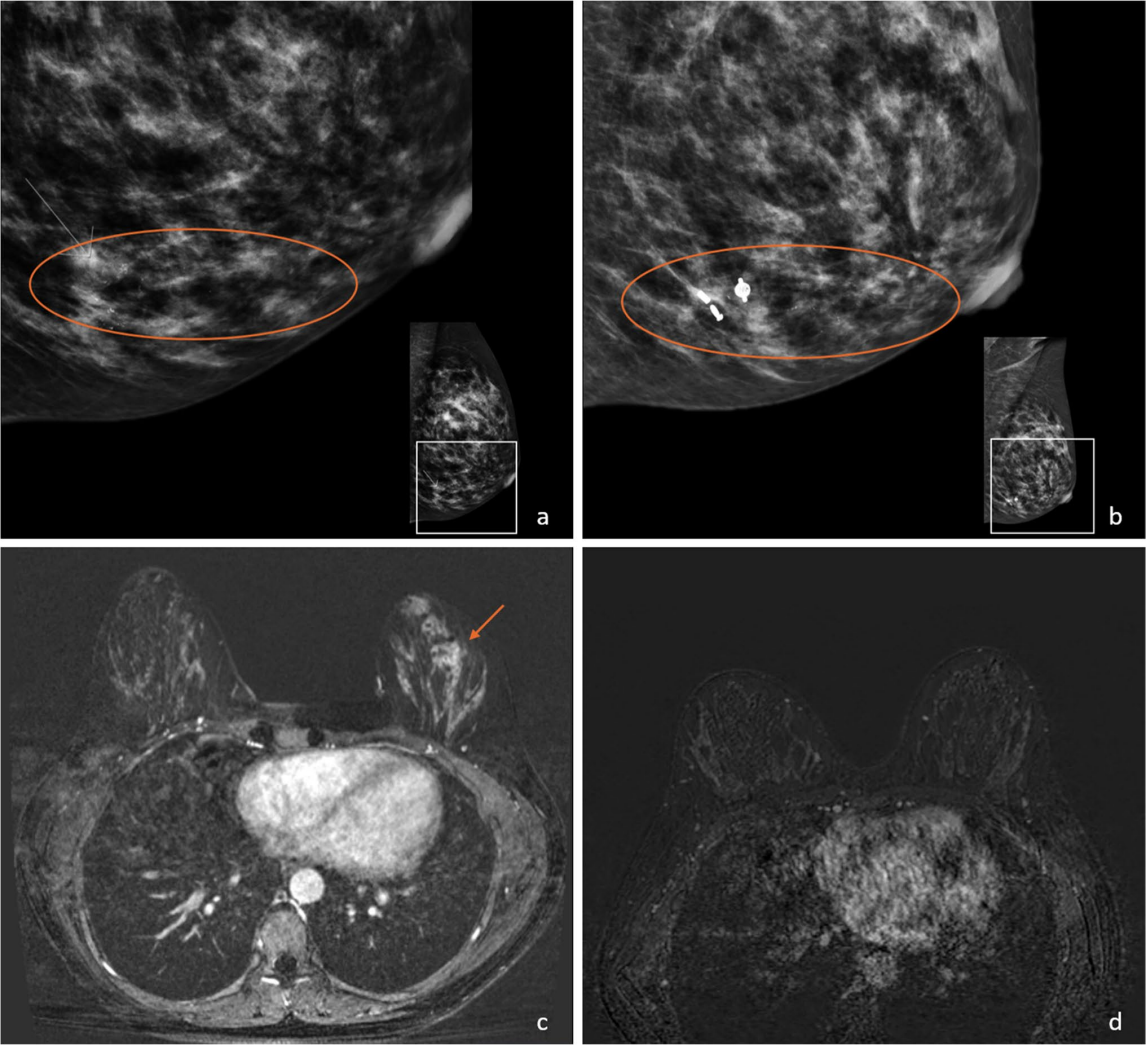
Pre- (a, c) and post-NST (b, d) mammography and MRI images of a patient with pCR of both IBC and DCIS. Fine pleiomorphic calcifications remained on mammography (a, b, orange circle), while NME (c, orange arrow) disappeared, classified as rCR on MRI
